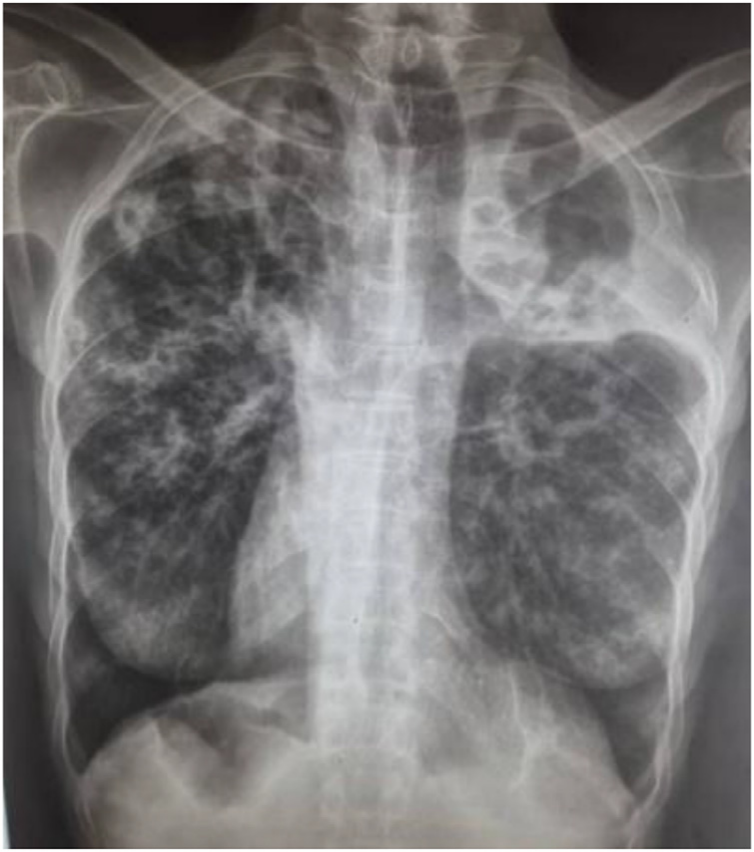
Chest X-ray showing bilateral alveolo-interstitial and cavitary lesions at the time of diagnosis in case 3.
